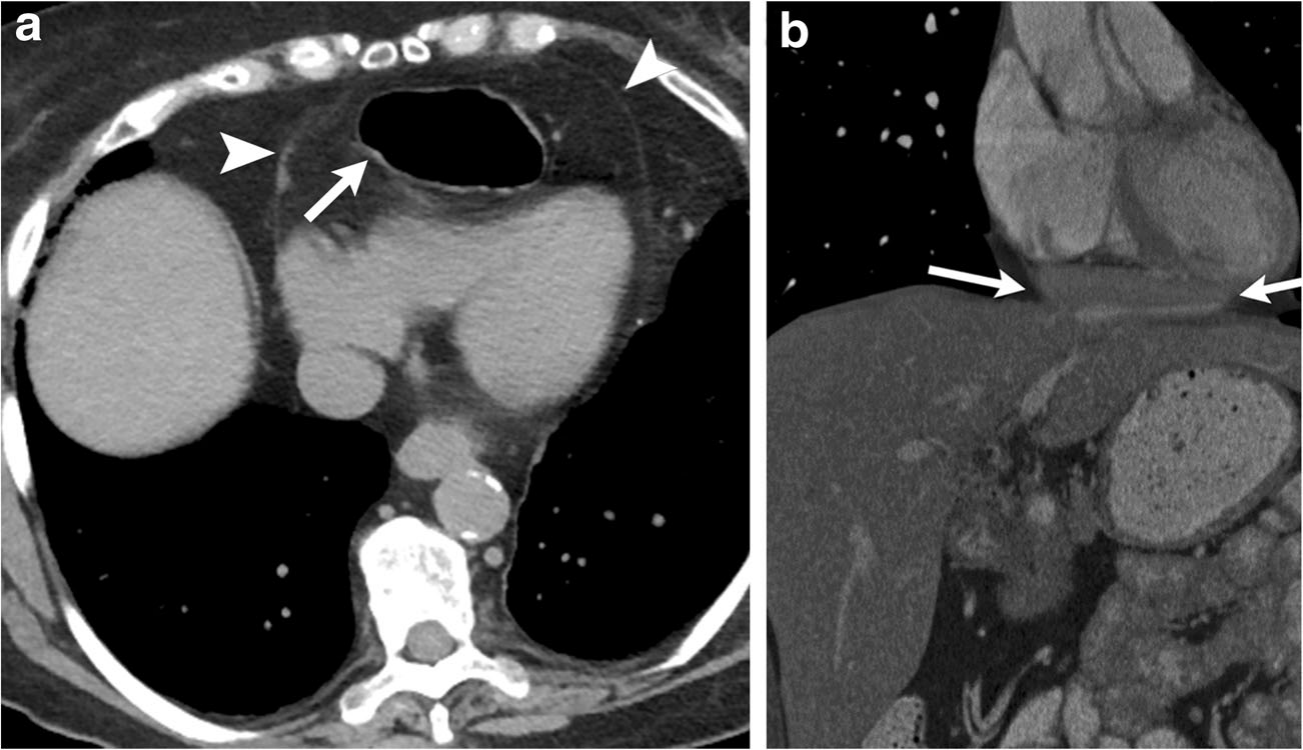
a A 44-year-old male with a recent history of blunt abdominal trauma, now presenting to the emergency department with upper abdominal pain. Axial CT with oral contrast identifies herniation of the stomach (white arrows) into the pericardium (arrowhead) with mild mass effect over the right ventricle. b A 56-year-old male with a remote history of blunt abdominal trauma and a recent diagnosis of Hodgkin's lymphoma. A pericardial mass was identified on a recent echocardiogram. Coronal CECT identifies herniation of the left lobe of the liver in the pericardium (white arrows). There is minimal mass effect over the right ventricle
